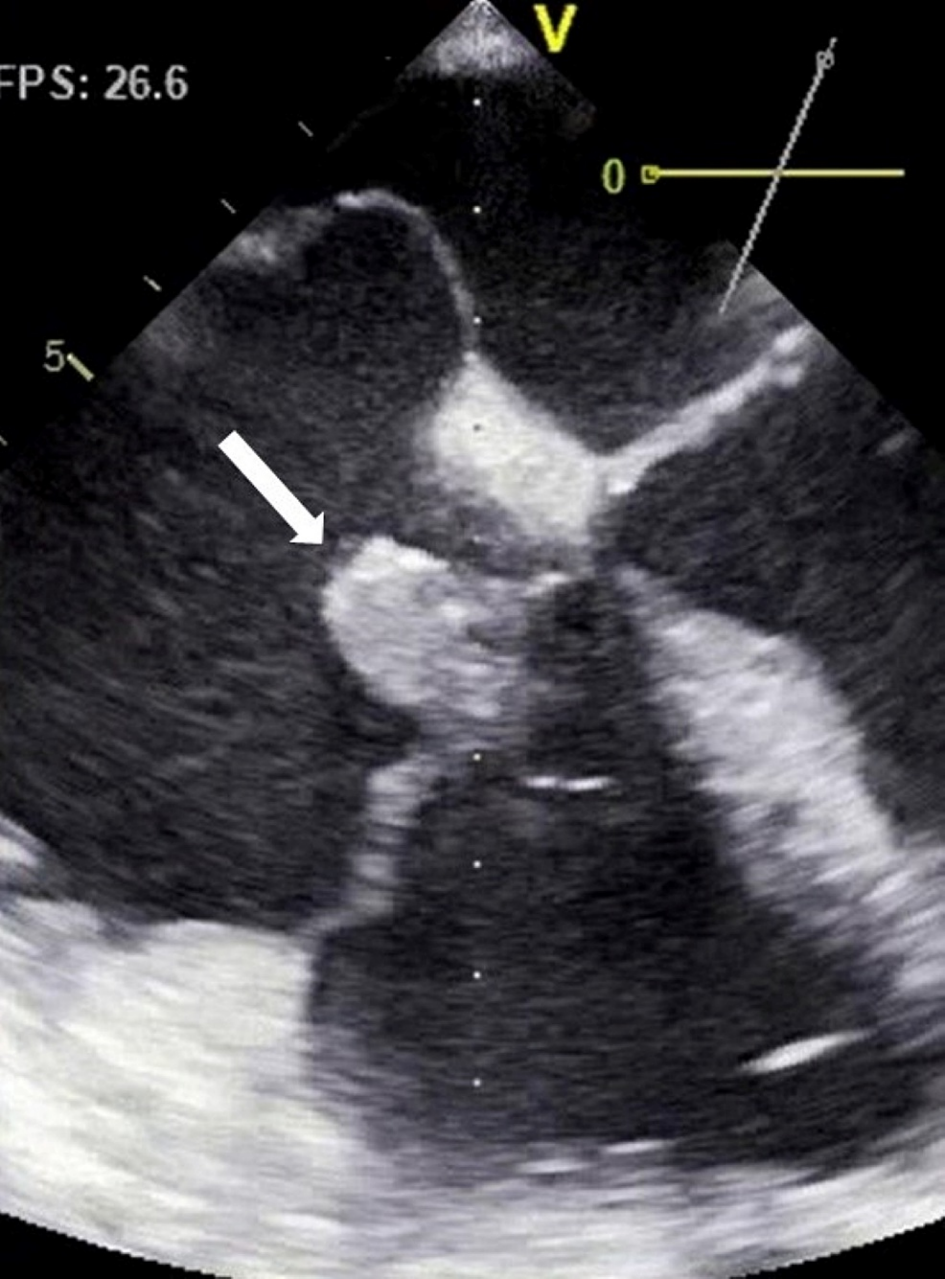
Transthoracic echocardiogram obtained during hospitalization, revealing a mobile tricuspid vegetation (white arrow), with dimensions of 22x18 mm.
